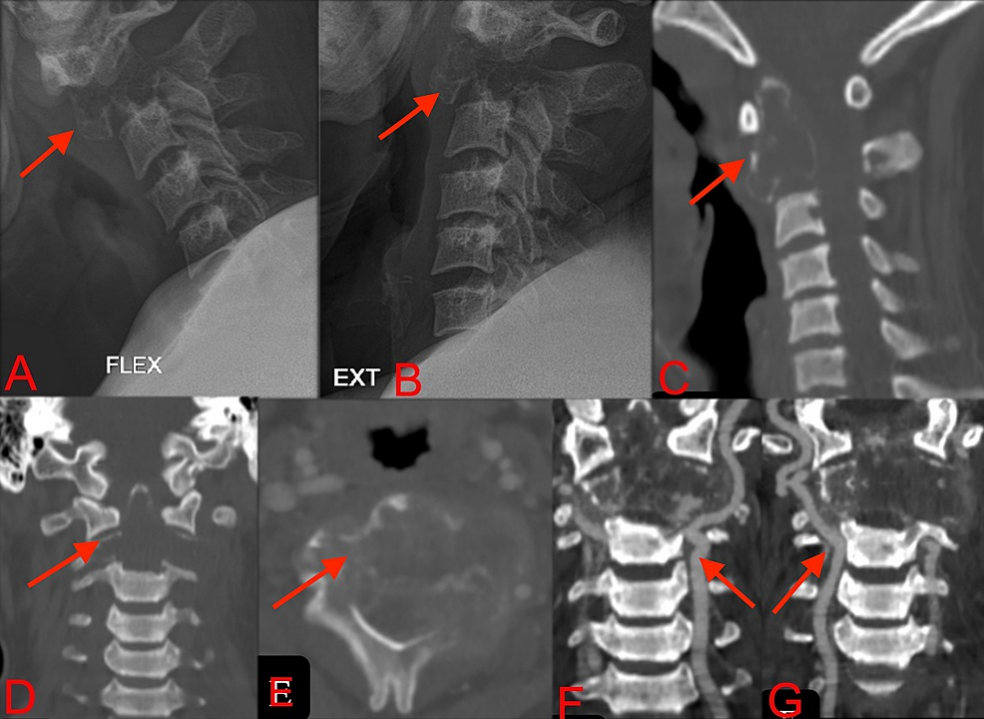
Lateral dynamic radiographs (A, B), CT sections- mid-sagittal (C), coronal (D), axial (E), and CT angiography (F, G) showed an expansile, lytic lesion involving odontoid process and anterior and posterior elements of C2 vertebra with cortical breach and sparing of bilateral vertebral arteries. CT: computerized tomography
